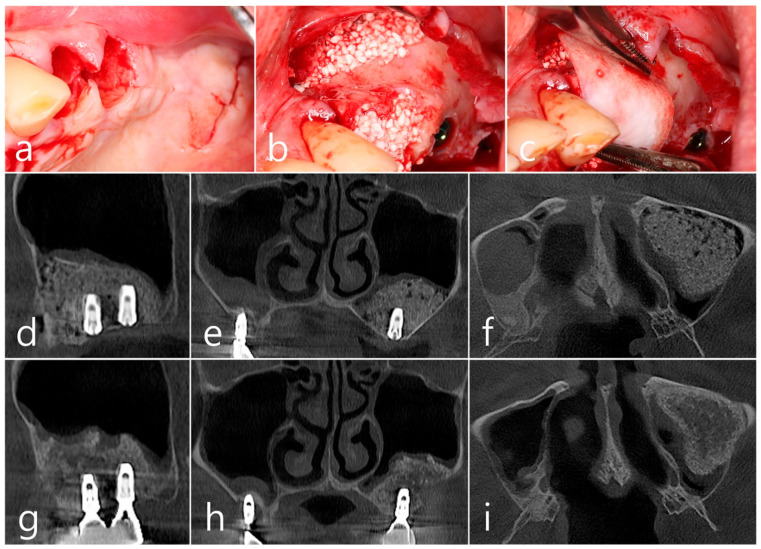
(a) Teeth #24 and #25 were extracted, midcrestal and vertical incisions were performed, and mucoperiosteal flaps were reflected; (b,c) A lateral sinus window was prepared, and sinus floor elevation was performed without membrane perforation. The bone graft substitute was delivered and covered with a resorbable collagen membrane. The flap was closed; (d–f) In the CBCT image taken immediately after the procedure, a well-filled bone graft substitute without displacement was observed; (g–i) Extensive bone resorption around #26 implant was observed in CBCT images taken 2 years after prosthesis delivery. The buccal cortical bone plate was lost, and bone resorption was present only within the sinus graft.
